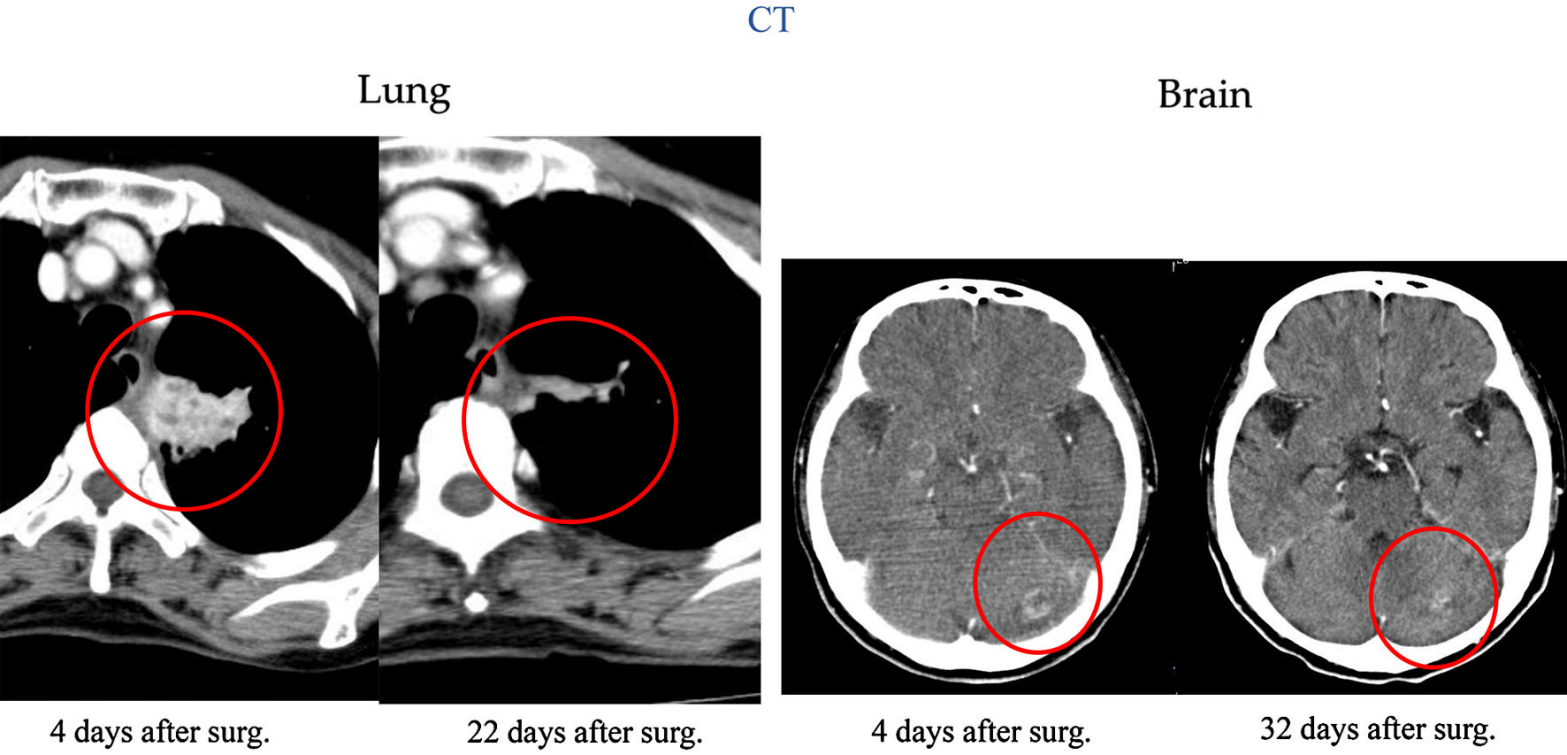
Follow-up CT. *Left* on postoperative day 4, an abscess was found at the left lung portal area, and by day 22, the abscess had decreased in size. *Right* a brain abscess, which was identified 1 day after surgery. By postoperative day 32, the abscess had decreased in size
